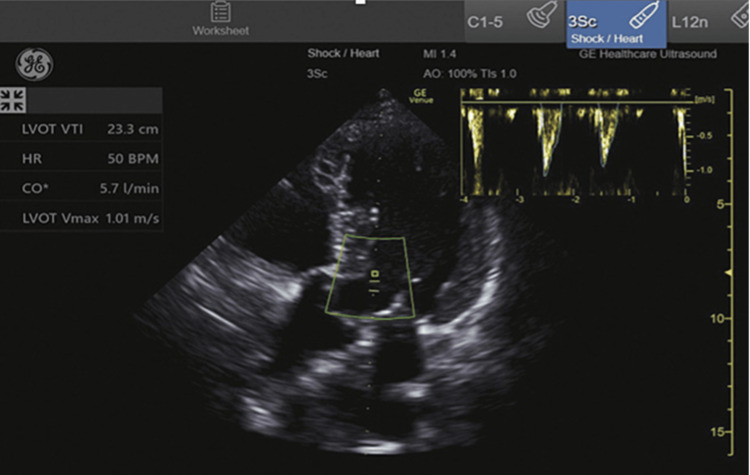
Point of care ultrasound with semi-automated software platform with LVOT VTI LVOT VTI: left ventricle outflow tract velocity time integral
